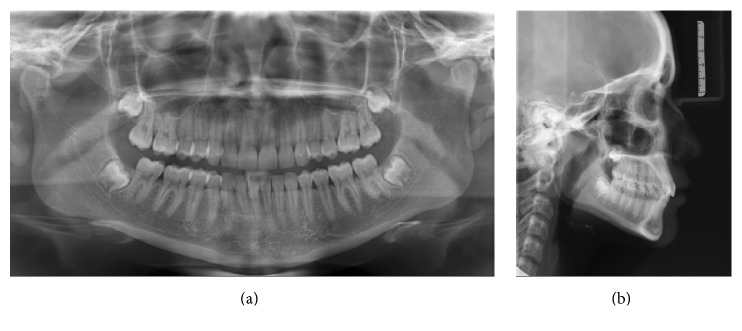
Final radiographs. In panoramic radiography, good positioning of all erupted teeth and parallelism of roots are observed (a); in teleradiography it is noted good maxillomandibular relationship as well as between the upper and lower incisors (b).
